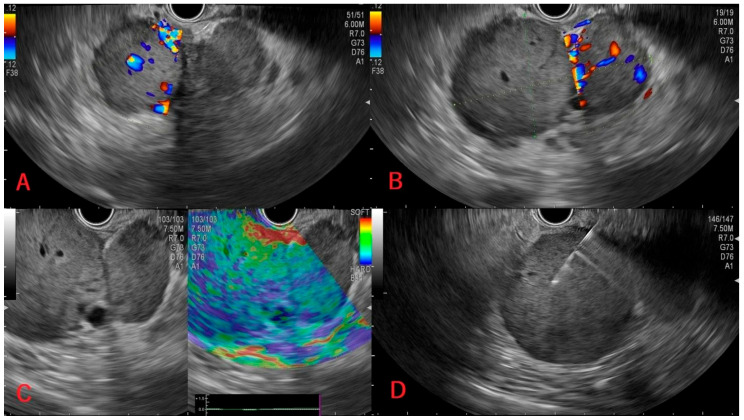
Case 3: Ultrasonography with an intense Doppler signal in both lobes of the pancreatic lesions (A,B), hard consistency of the pancreatic tumor (C), and tissue sampling through EUS (D).
